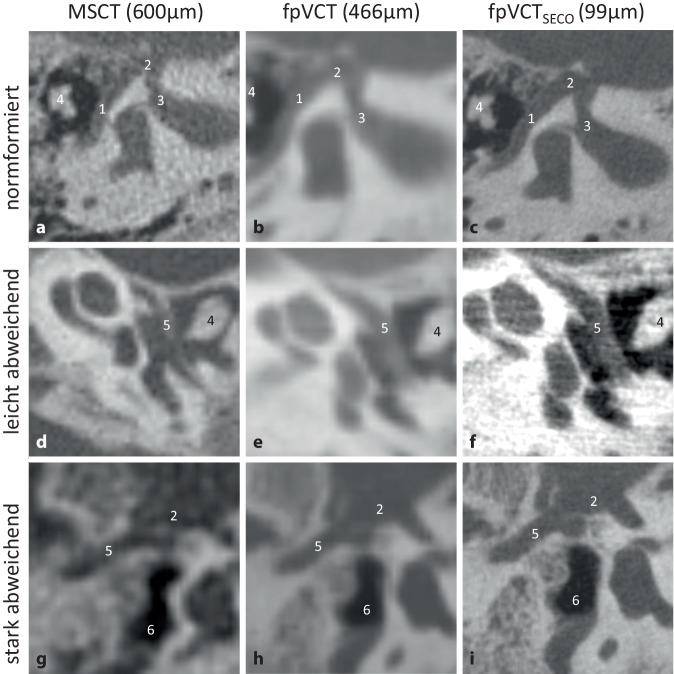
Visualisierung des Verlaufs des Nervus facialis. Ein repräsentatives Bild mit MSCT (600 µm Schichtdicke) ist in a, eine Darstellung von fpVCT (466 µm Schichtdicke) in b und fpVCT_SECO_ (99 µm Schichtdicke) in c gezeigt. d–f zeigen einen leicht abweichenden Verlauf des N. facialis durch die Paukenhöhle. g–i zeigen einen stark abweichenden Verlauf des N. facialis. *1* Canalis nervi facialis, *2* Ganglion geniculatum, *3* Meatus acusticus internus, *4* Malleus-Incus-Komplex, *5* dysmorpher Verlauf des N. facialis, *6* dysmorphe verkleinerte Paukenhöhle. *MSCT* Multislice-Computertomographie, *fpVCT* „flat-panel-volume computed tomography“, *fpVCT*_*SECO*_ sekundäre Rekonstruktionen der fpVCT
